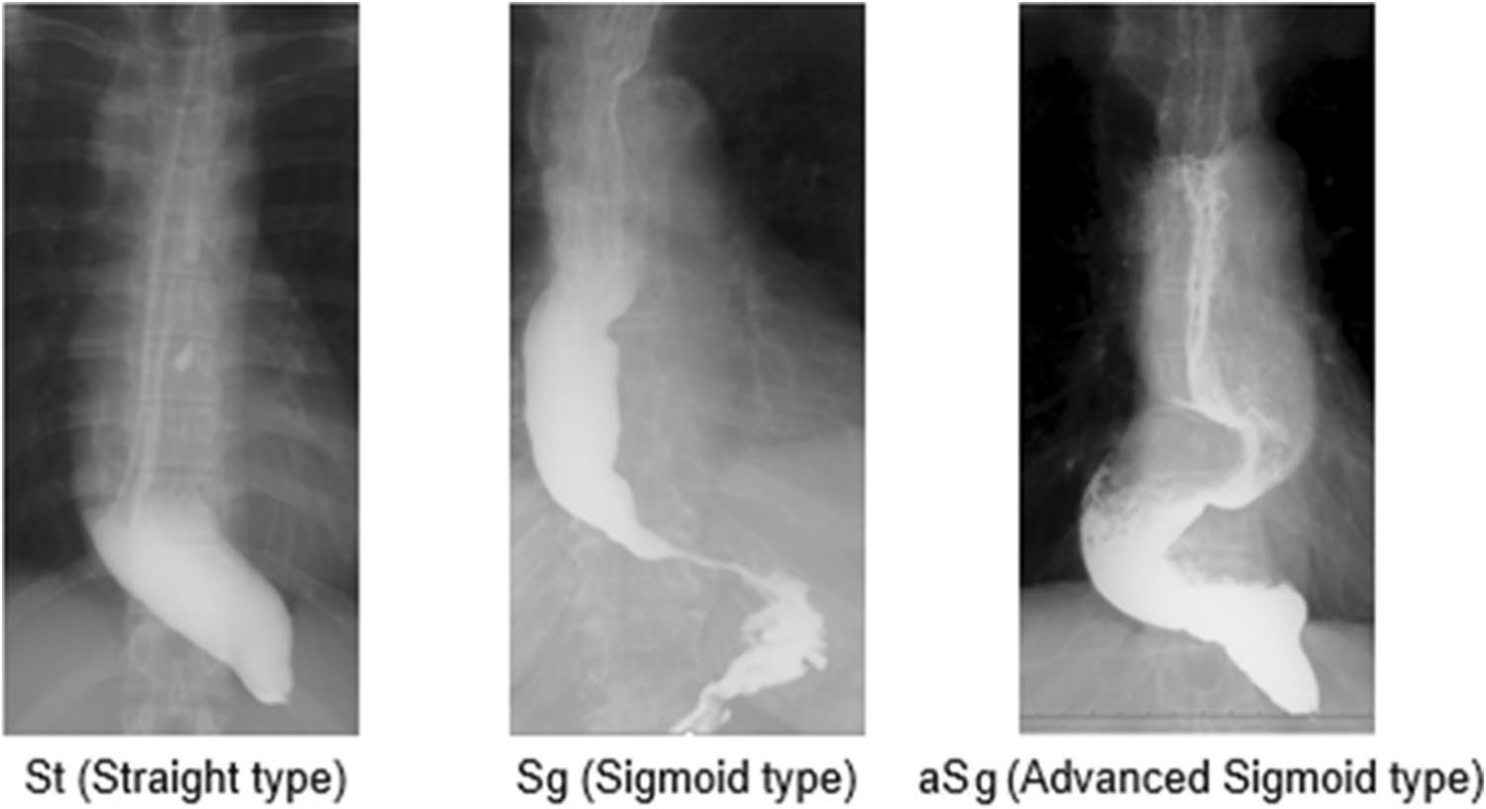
New Japanese X-ray classification system for esophageal achalasia was revised in 2012. Esophageal achalasia is classified into 3 types, St (Straight type), Sg (Sigmoid type) and aSg (Advanced Sigmoid type), based on the X-ray findings. If there is a bending of the esophagus, draw a straight line to the esophagus long axis direction. Determine the angle *α* at the intersection of two straight lines. (St: *α* ≥ 135°, Sg: 90° ≤ *α* ≤ 135°, aSg: *α* < 90°)
